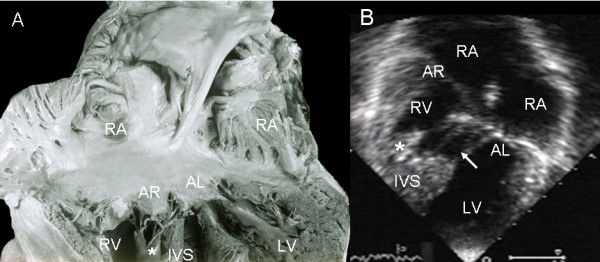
Atrioventricular septal defect with common atrioventricular valve in dextroisomerism. (A) Note the mixed insertion of the anterior leaflets in the crest of the ventricular septum (Rastelli type A) and a papillary muscle (asterisk) adjacent to the ventricular septum (Rastelli type B). (B) Echocardiographic 4-chamber image in dextroisomerism shows a similar insertion.
